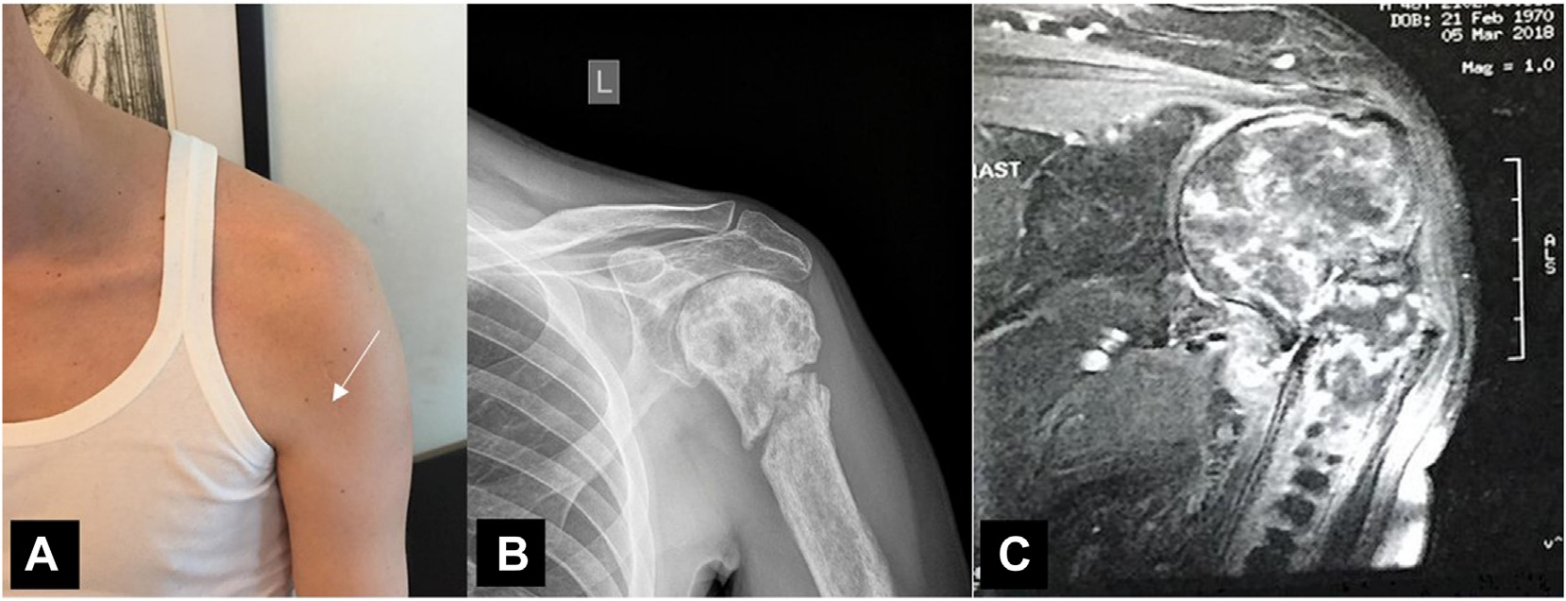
(A) Severe muscle atrophy of the anterior (*arrow*) and middle deltoid, (B, C) Plain X-ray and MRI scan showing extensive avascular necrosis and signs of postradiation osteoarthritis, along with nonunion of the fracture at the surgical neck and also severe tendinopathy and atrophy of the muscles of the rotator cuff. *MRI*, magnetic resonance imaging.
